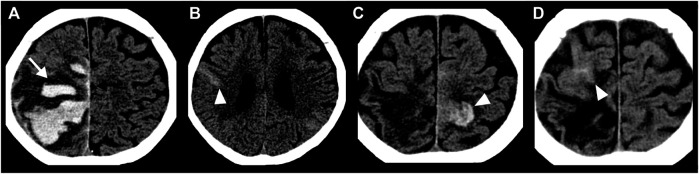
Head CT images of an 84-year-old woman with CAA showing repeated non-traumatic intracranial hemorrhage over four months. (A) ICH presenting finger-like projections (white arrow) with SAH extension (black arrow). (B) Acute convexity SAH (arrowhead) at two months later than A. (C) Acute convexity SAH (arrowhead) at three months later than A. (D) Acute convexity SAH (arrowhead) at four months later than A.
